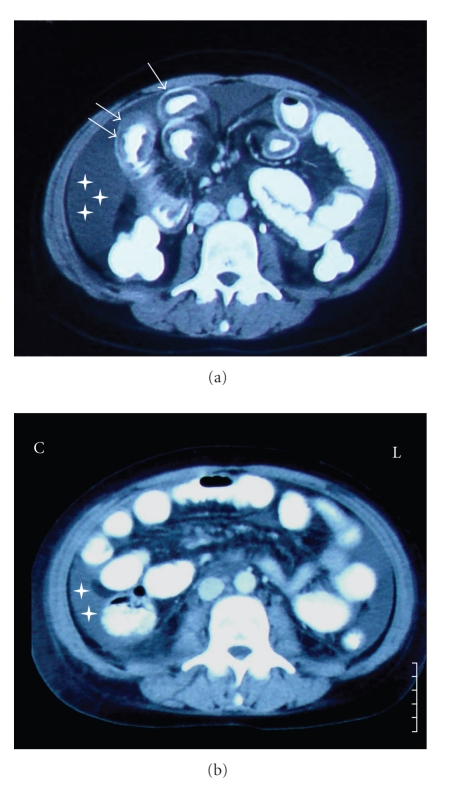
Computed tomography scan of the abdomen performed. (a) On day 3 of the patient's admission, 4 days after initiation of symptoms. Arrows: thickening/dilatation of the intestinal wall, stars: ascitic fluid. (b) Four days after initiation of treatment, with marked improvement of the intestinal morphology, and moderate reduction of the amount of ascitic fluid.
